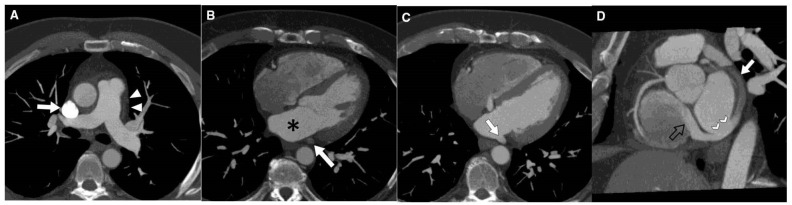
Contrast-enhanced cardiac CT images revealing unroofed coronary
